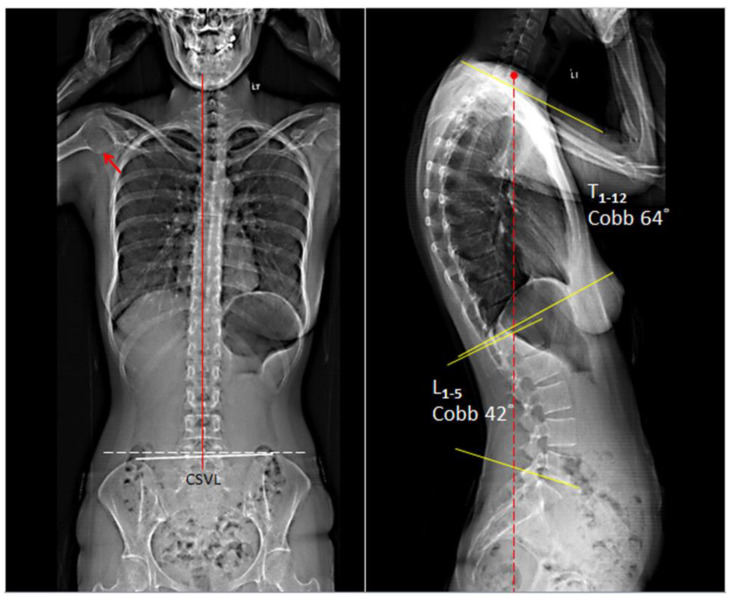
EOS^®^ radiographs demonstrating degenerative spondylosis of the lower cervical spine with osteophytes at posterior C5 and C6, C7/T1 disc-space narrowing, straightened cervical lordosis and thoracic hyperkyphosis, and cystic change of the right humeral head (red arrow). In general, the average angle of thoracic kyphosis and lumbar lordosis is 43.55° ± 6.44 and 32.42° ± 6.29, respectively. CSVL: central sacral vertical line (red line).
